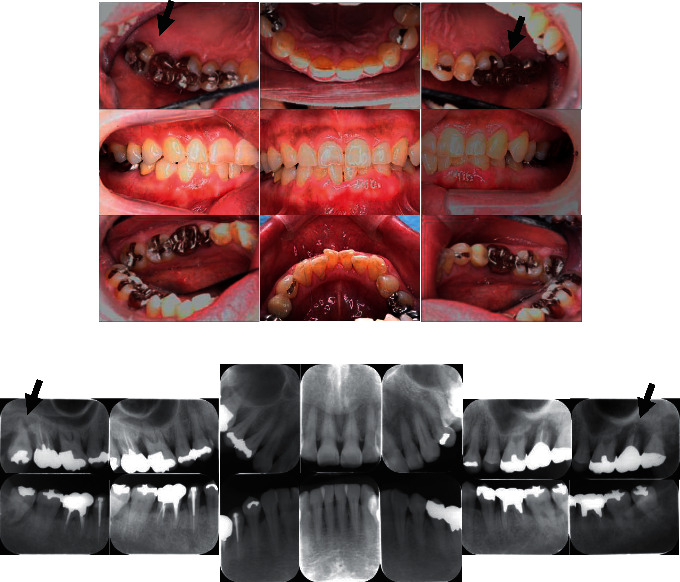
Appearance of the patient's periodontal tissues at the first visit (a) and radiograph of the periodontium (b). Note the severe alveolar bone resorption and so-called “floating teeth” due to severe periodontitis (b) and moderate gingival inflammation (a). “Floating teeth” were indicated by arrows. In addition, many metal restorations are observed.
